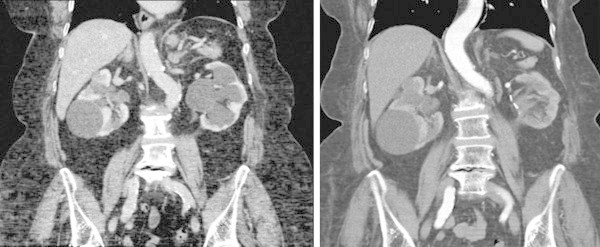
CT scan. On the left: left UPJ obstruction and hydronephrosis and on the right: disappearance of left hydronephrosis after surgery.
